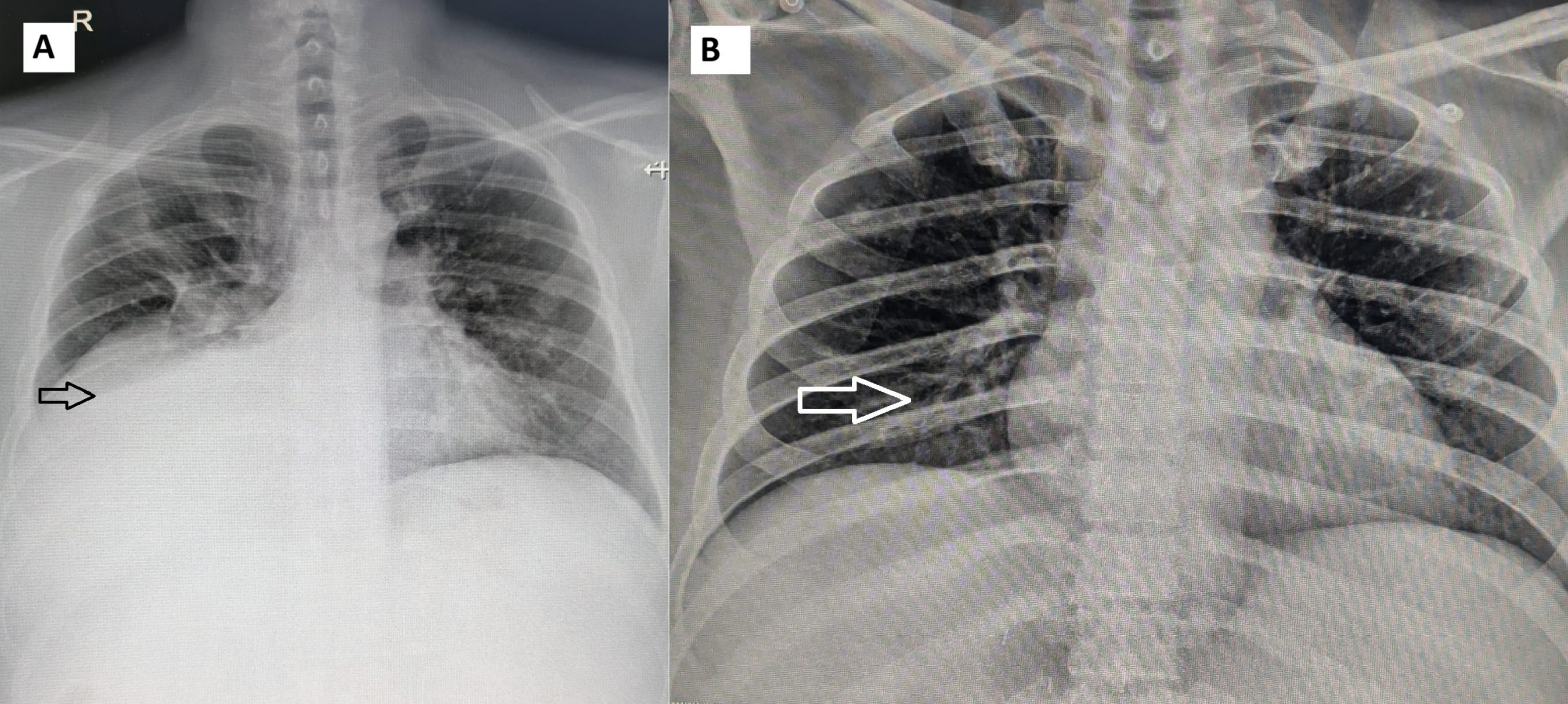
Case 3 (A) Chest X‐Ray showing right‐sided pleural effusion, (B) Follow‐up chest radiograph showing complete radiological resolution.
